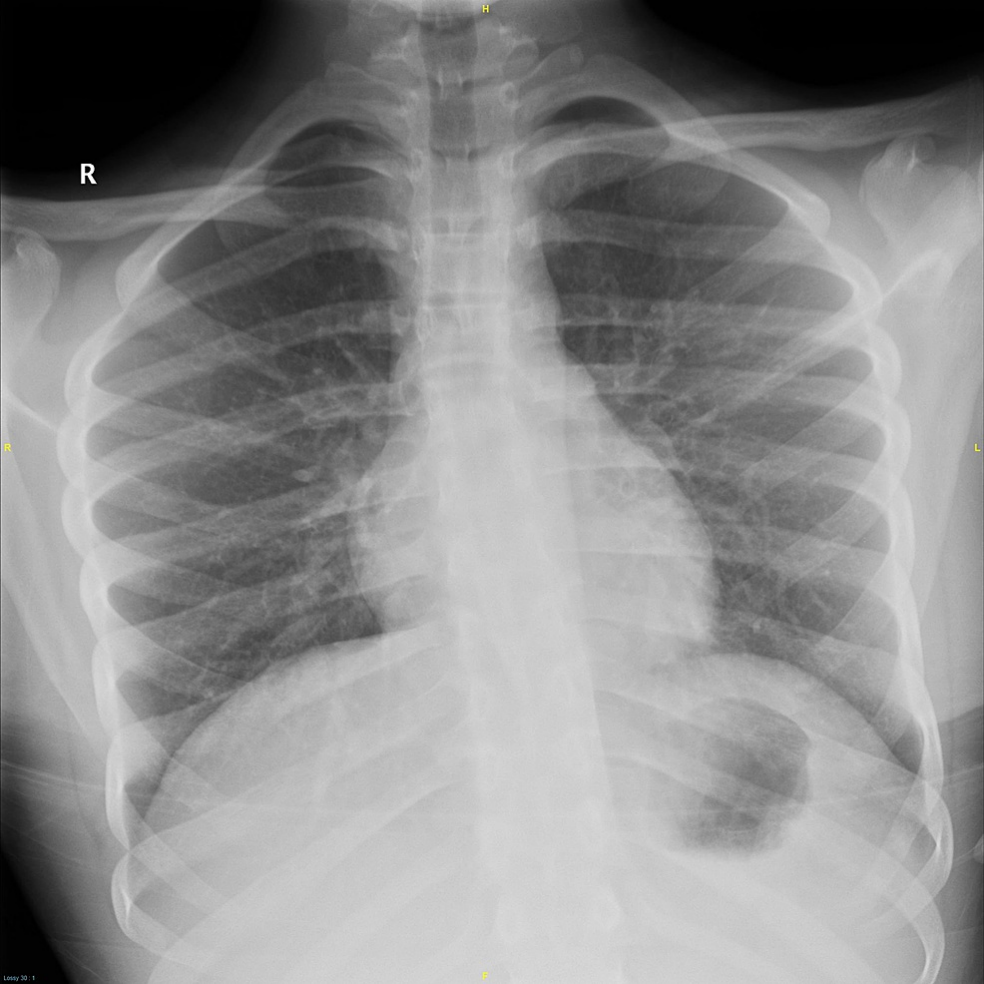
Chest x-ray on admission was normal.
